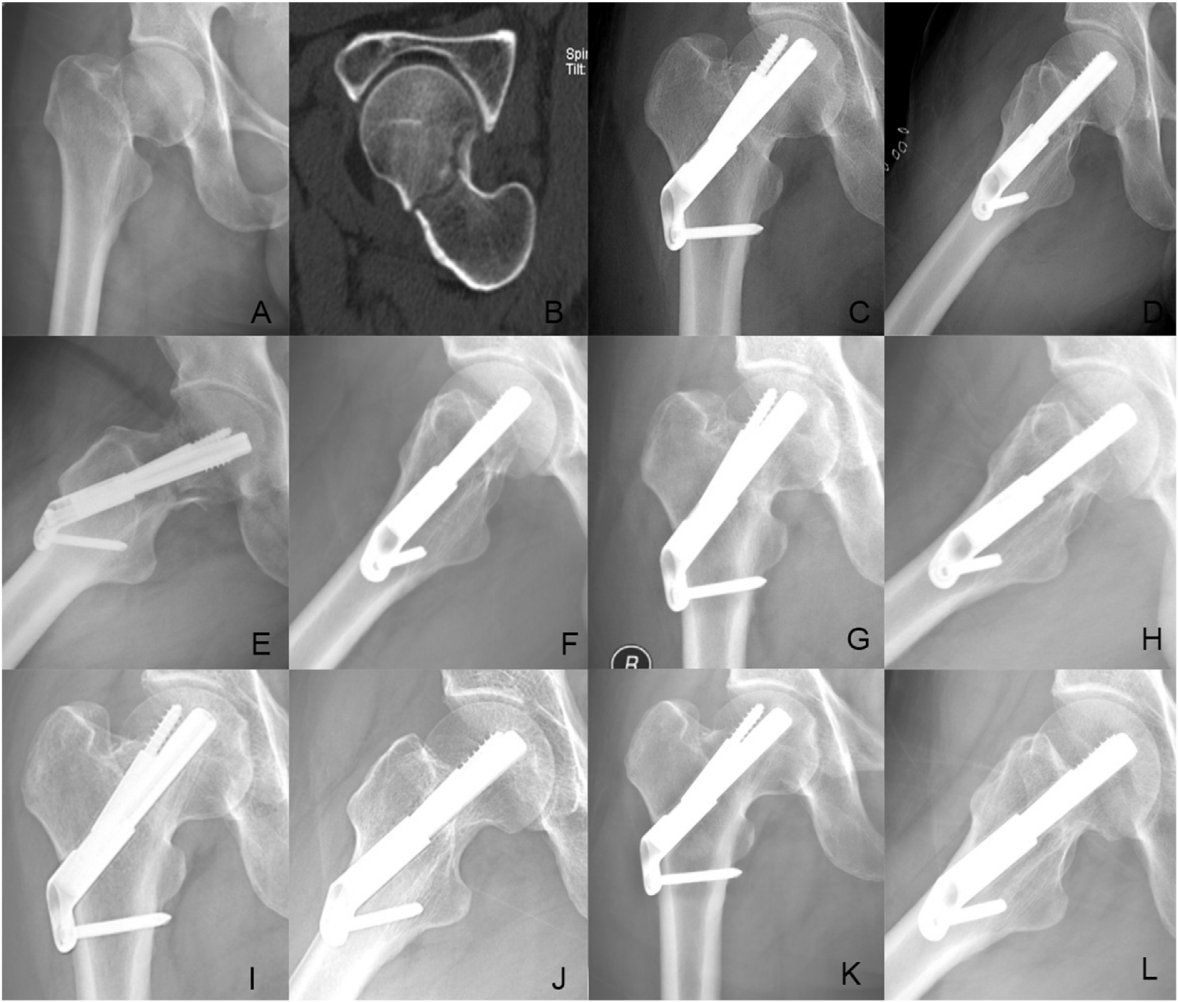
Case presentation: a 42 years old man with right femoral neck fracture fixed with FNS. (A,B) Preoperative anteroposterior radiograph and CT scan showed the right FNF with posteromedial cortex fracture. (C,D) The anteroposterior and lateral X-ray with FNS 1 day after surgery; (E,F) The anteroposterior and lateral X-rays 1Dmonth after surgery; (G,H) The anteroposterior and lateral X-rays 3 months after surgery; (I,J) The anteroposterior and lateral X-rays 6 months after surgery; (K,L) The anteroposterior and lateral X-rays 12 months after surgery.
